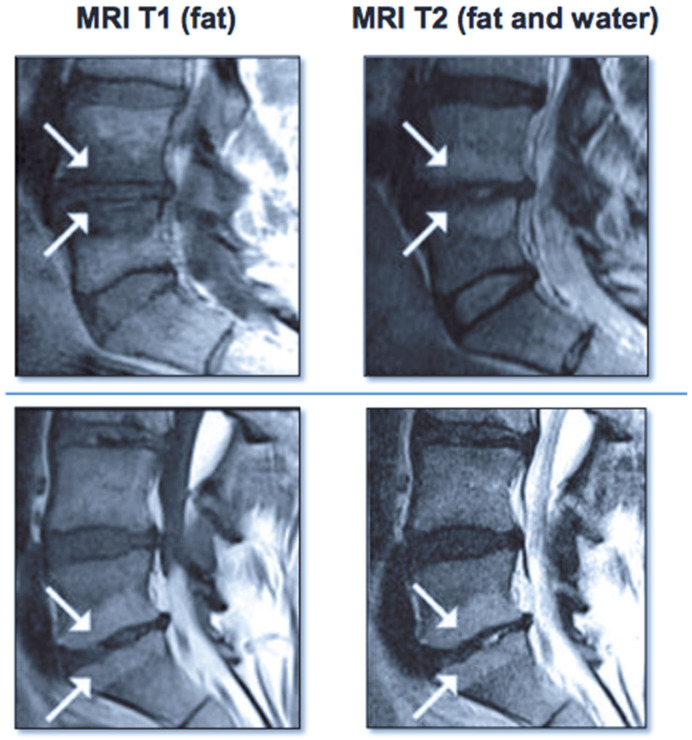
Images on the left show Modic type I changes in MRI with hypo-intense T1 signal Images on the right show Modic type II changes with hyper-intense T1 and T2 signals.
